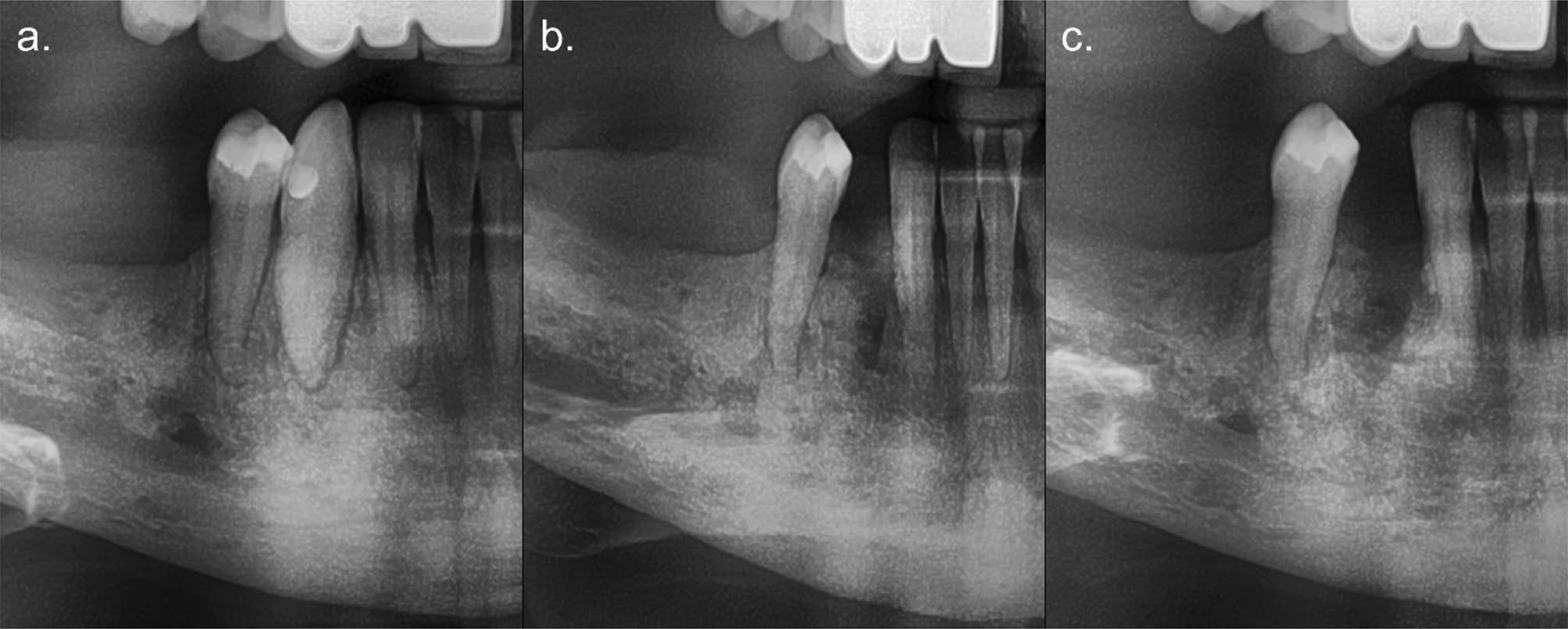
Cropped panoramic radiographs of a 67-year-old female in treatment with denosumab, showing tooth 43 two months before extraction (a), and ten (b) and eighteen months after (c). This site had absence of periapical lesion and angular bone defect, but presence of sclerosis and widening of the periodontal ligament space. Osteonecrosis was diagnosed eight weeks after surgery, as the site did not heal. Persistence of the alveolar socket and sclerotic pattern can be seen postoperatively.
